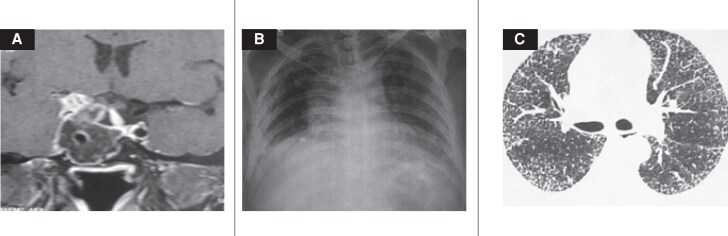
(A) Coronal T1-weighted MRI showing a sellar lesion with right parasellar extension consistent with a prolactin-secreting pituitary adenoma. (B) Chest radiograph demonstrating reticular opacities in the lower lung fields. (C) HRCT of the thorax revealing reticular thickening and honeycombing, suggestive of a usual interstitial pneumonia pattern. Abbreviations: HRCT, high-resolution computed tomography; MRI, magnetic resonance imaging.
